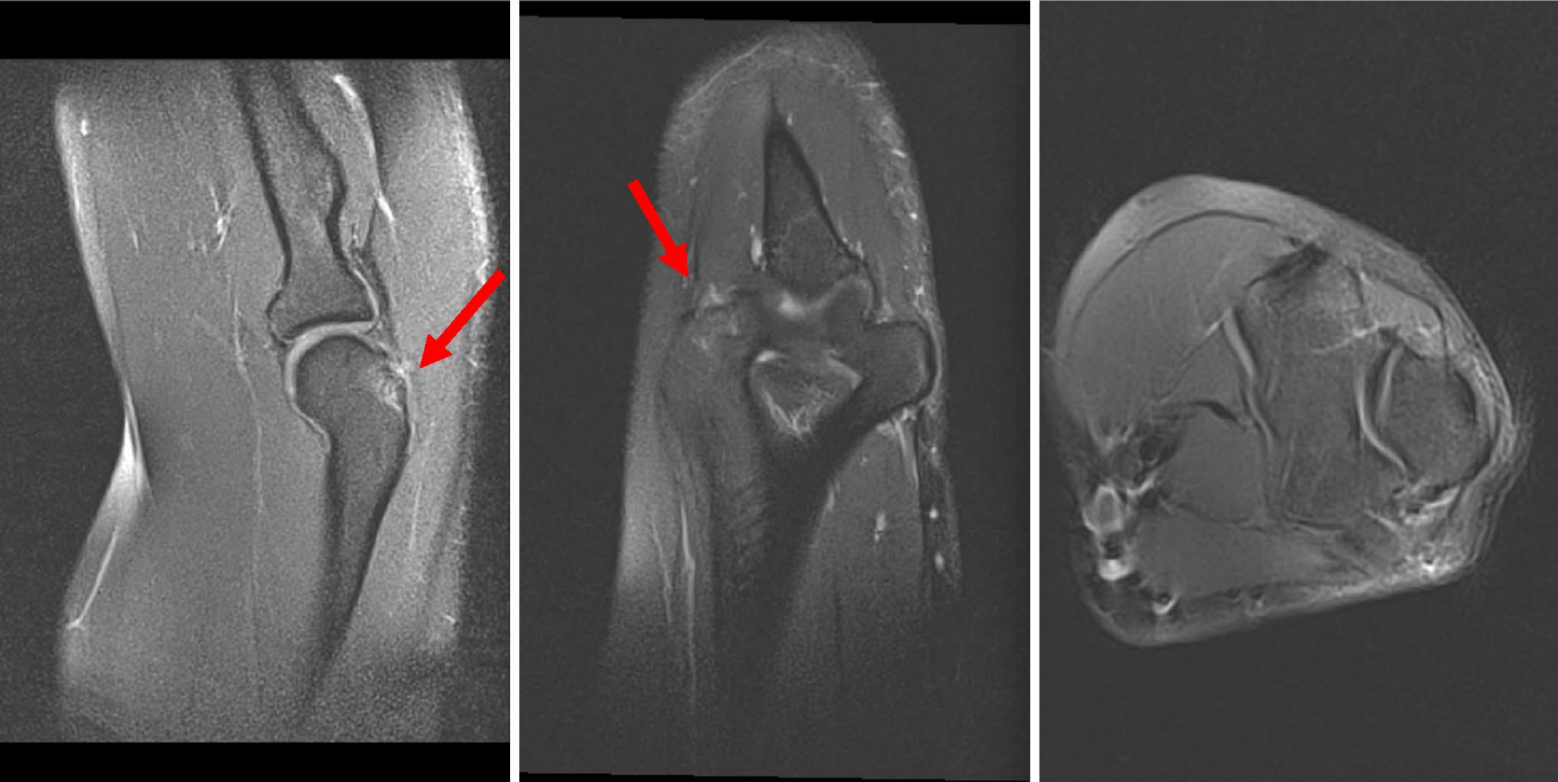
MRI of the right elbow of patient no. 5 at first presentation (the arrow marks the bone marrow edema at the posterior aspect of the elbow)
